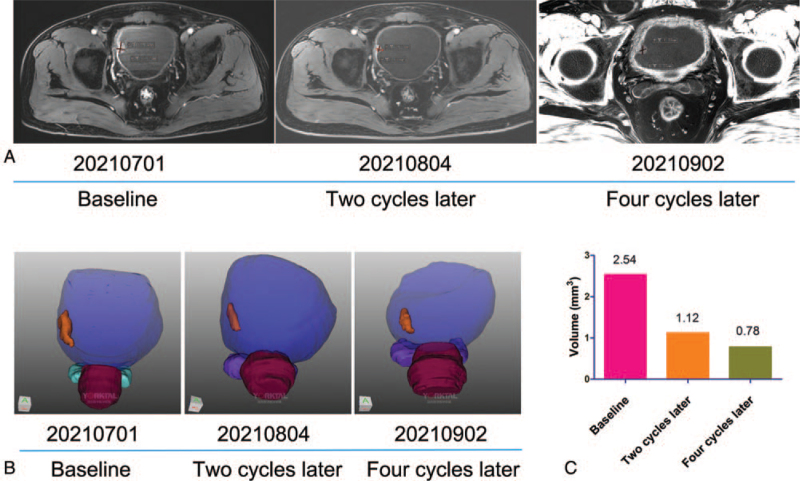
Time line of treatments of the patient and changes in MR scan and MRI 3-dimensional image reconstruction.
